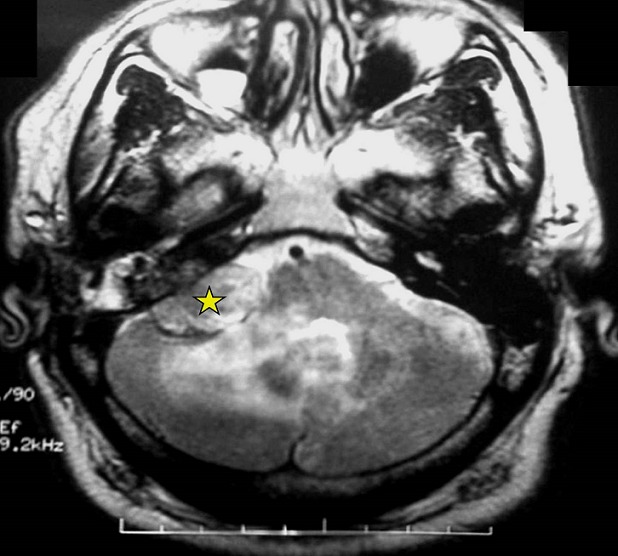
Cerebral axial T2-weighted magnetic resonance imaging showing a well circumscribed multi-lobulated extra-axial mass in the right cerebellopontine angle with cerebellar compression
